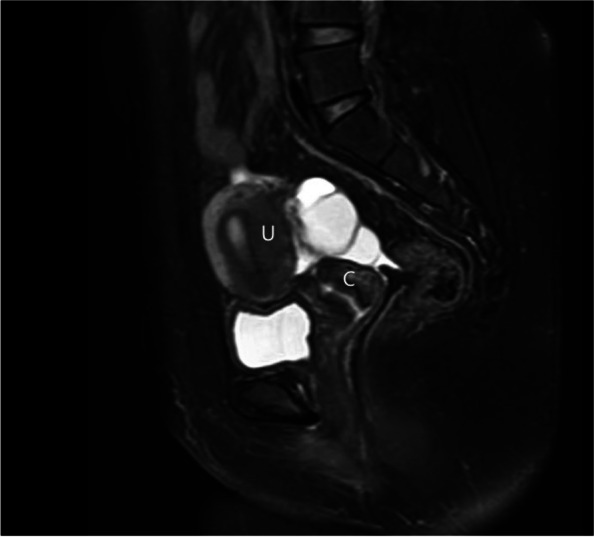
MRI examination showed a complete separation of the cervix (C) from the uterine corpus (U)
